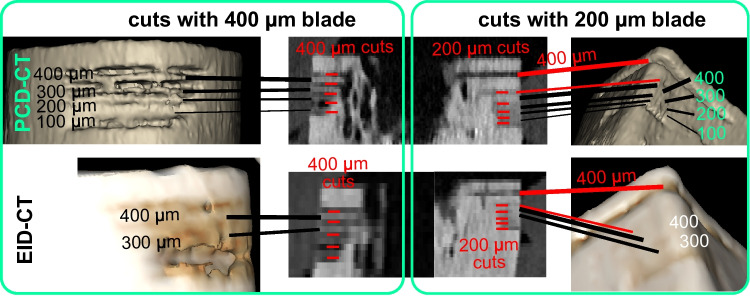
3D models generated from PCD (top) and EID (bottom) CT image series, showing bony displacements (lamellae) generated with a blade width of 400 µm (left) and 200 µm (right). Additionally, a single incision with 400 µm, separated by 1 mm, is shown on the right
